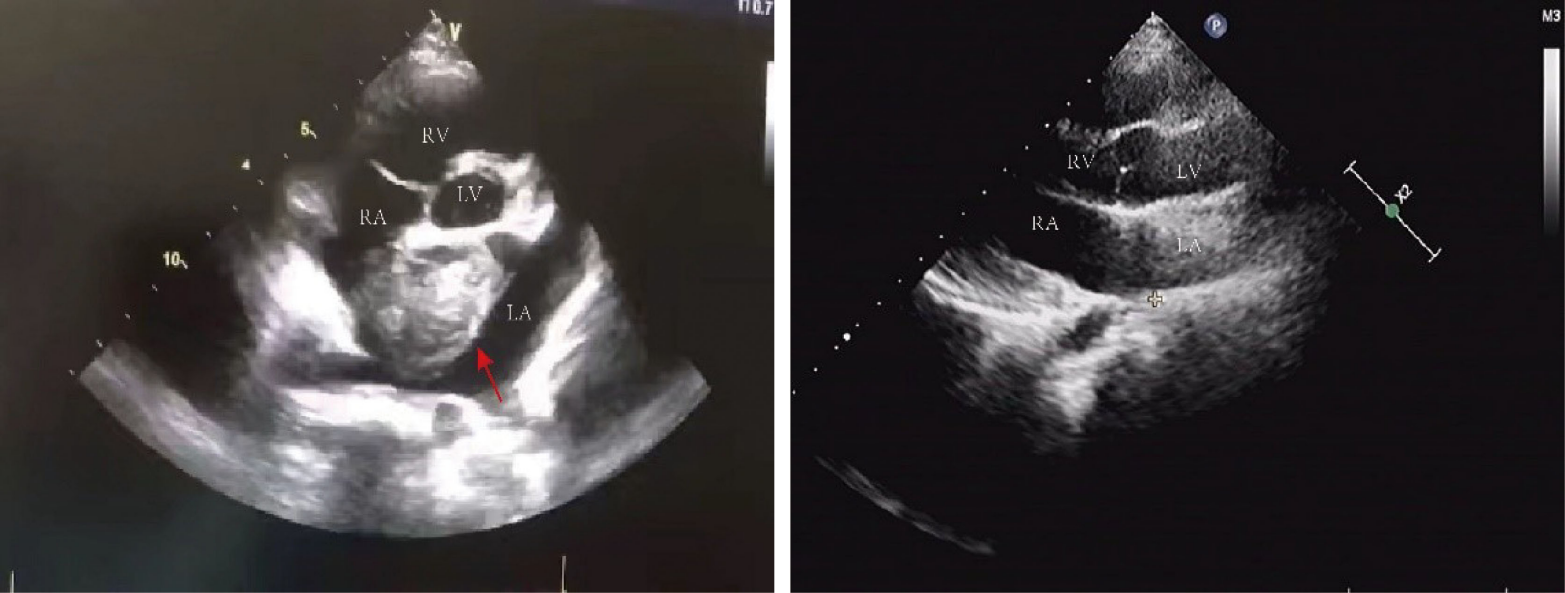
Echocardiography: there was a mass of 36 mm X 44 mm in size in the left atrium with roughly uniform echogenicity (red arrows pointing to the tumor in the left picture). The post-operative ultrasound is shown on the right picture. LV, left ventricle; RV, right ventricle; LA, left atrium; RA, right atrium.
